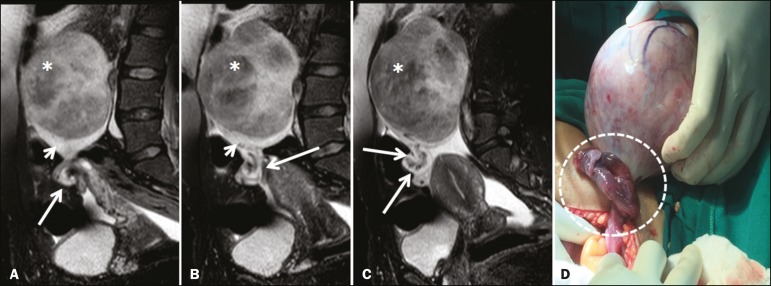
A–C: Sagittal fat-suppressed T2-weighted SPIR MRI sequences. Note the voluminous mass within the left ovary (asterisks), resulting in adnexal torsion, characterized by the whirlpool sign, with associated flow voids (long arrows), indicative of vascular torsion. The affected ovary (asterisk) is elevated and shows peripheral edema (short arrows). D: Intraoperative image showing the twisted, slightly ischemic adnexal pedicle (dashed outline) and the ovarian lesion that was later identified as a fibrothecoma.
